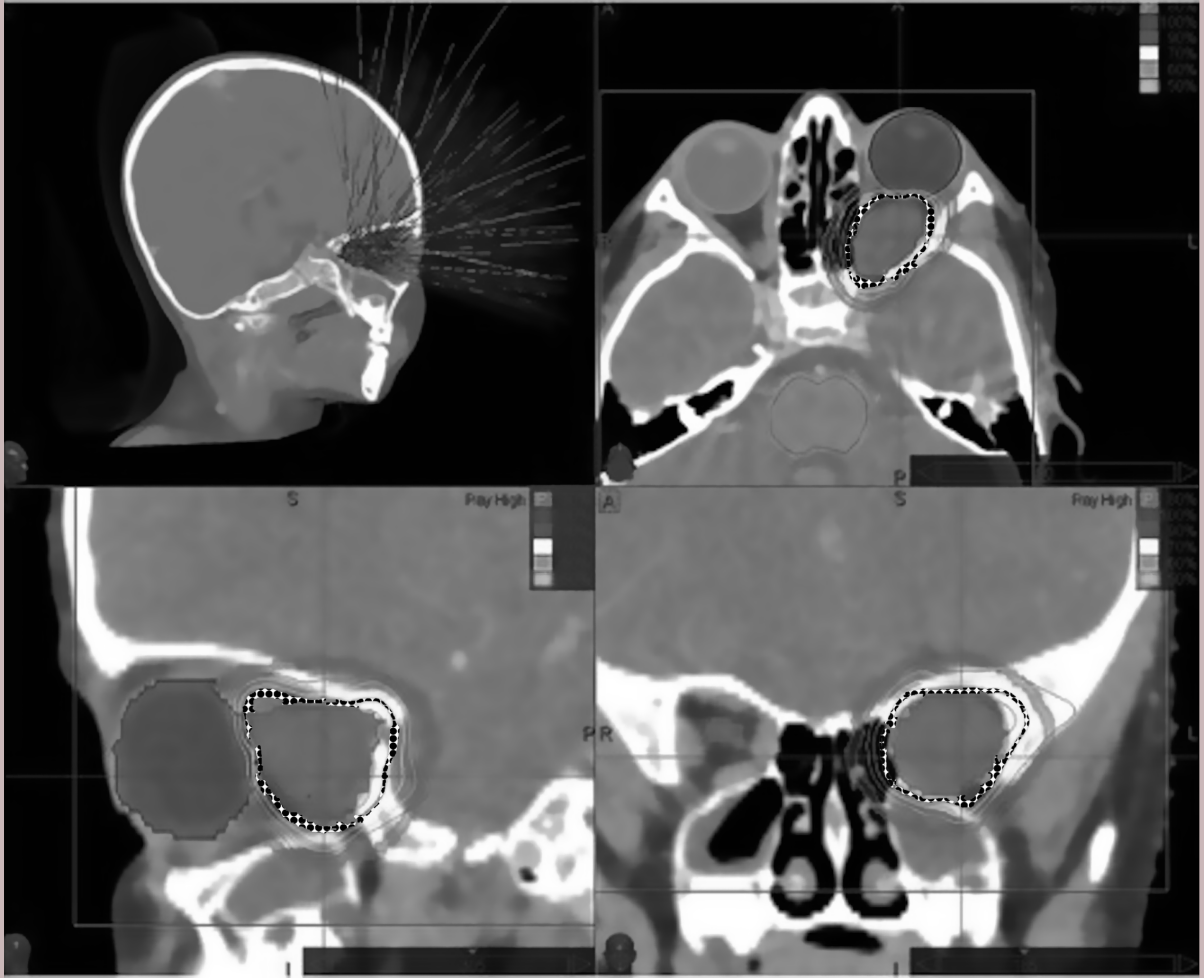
The CK plan of a patient with primary ONSM surrounding the left optic nerve. The left-upper image shows the active and passive beams directed to the PTV. The right upper, left lower, and right lower images shows the axial, sagittal, and coronal sections of the planning CT, respectively. The dose is prescribed to the 80% isodose shown in an dotted interrupted line around the PTV.
